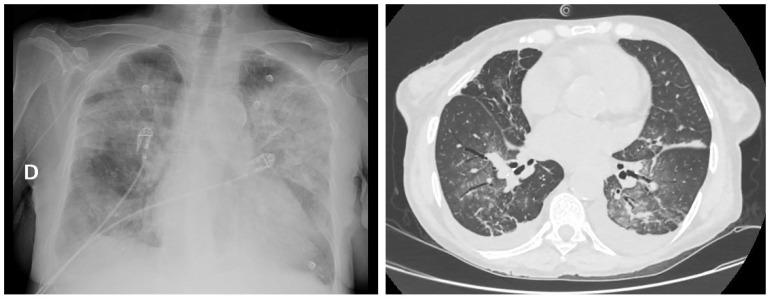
Chest X-ray and TC scan documenting bilateral pulmonary infiltrates and pleural effusion during ATRA differentiation syndrome (Carestream Vue Motion Version 12.1.5.9529 © Carestream Health, Inc., Rochester, NY, USA, 2021). D: RIGHT side.
